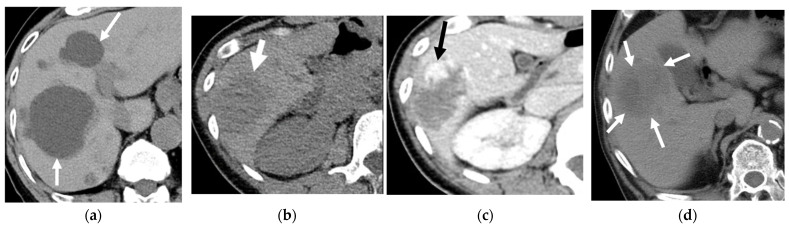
Incidental liver lesions in low-dose computed tomography (LDCT) scans. (a) Simple hepatic cysts (arrows). (b) A LDCT scan of a 40-year-old female showing a 4.9 cm hypodense mass in the right lobe liver (arrow). (c) A dynamic contrast-enhanced CT of the liver was arranged. The CT scan reveals peripheral nodular and centripetal enhancement, suggesting hemangioma (arrow). (d) A 5.3 cm hypodense mass (arrows) in the LDCT scan of a 56-year-old male was ultimately proven to be hepatocellular carcinoma.
